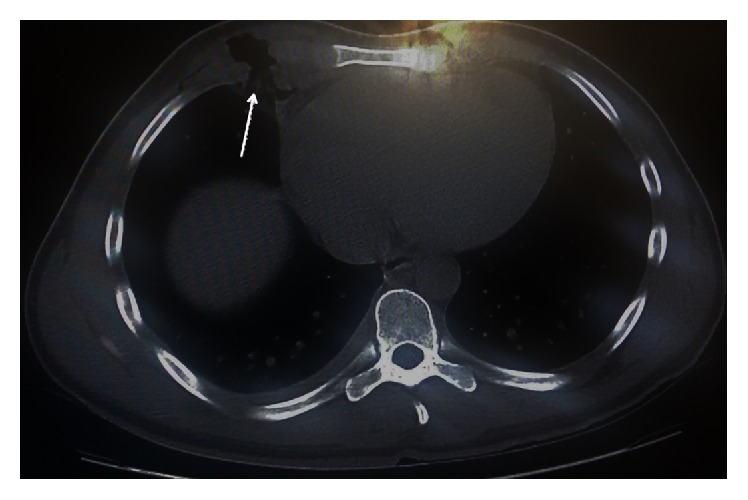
Axial contrast-enhanced CT of the chest. Note the disruption in the thoracic cavity with lung anterior herniation due to fractured ribs (arrow).
